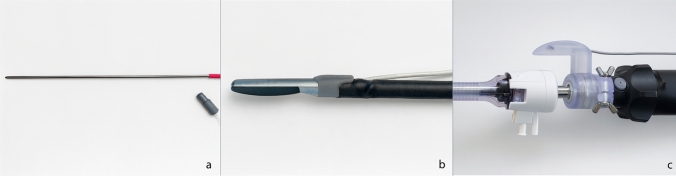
Tracked surgical instruments included a a laparoscopic pointer, b a laparoscopic ultrasound probe, and c a 30° laparoscope
